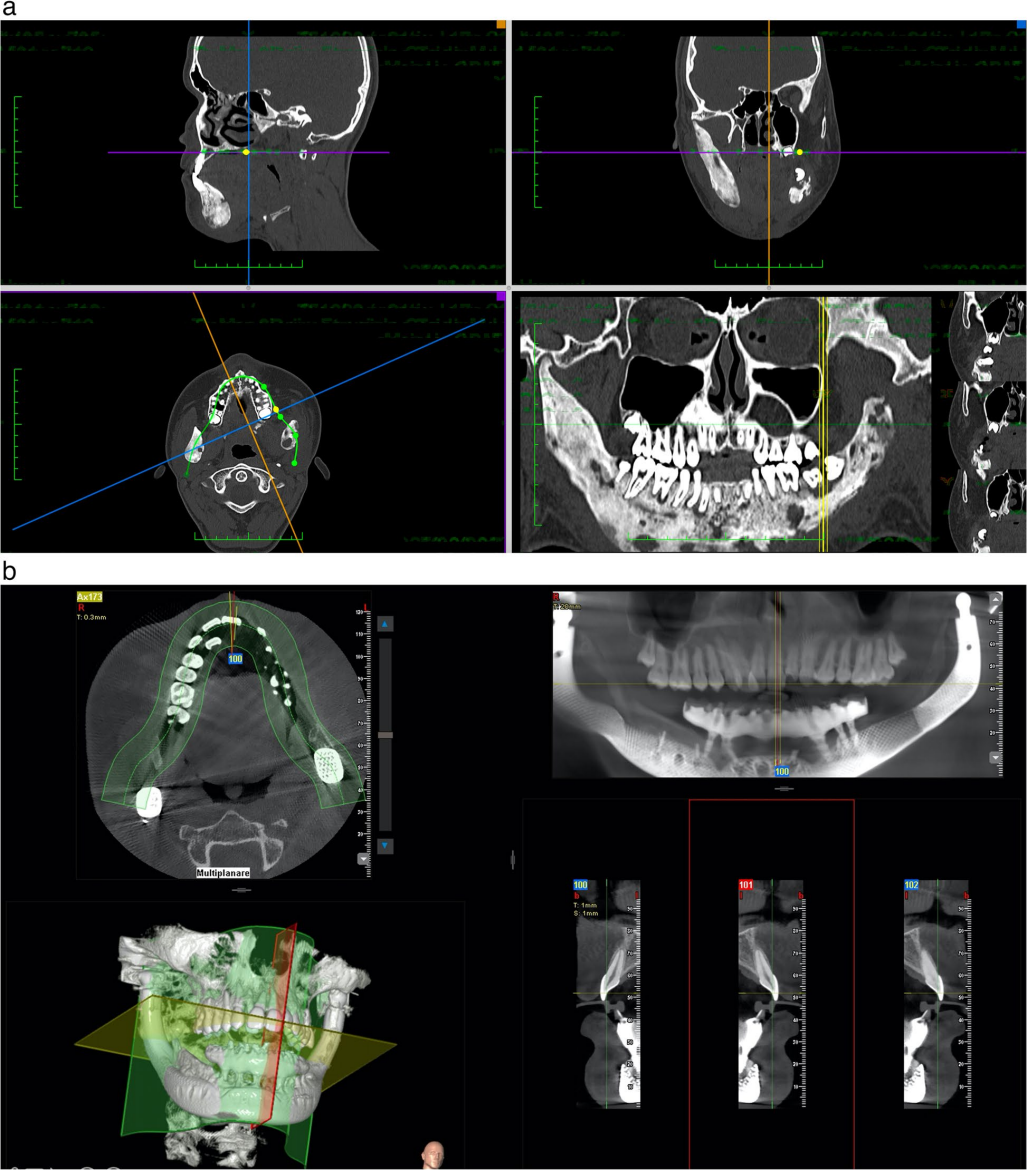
a Pre-operative CT scans. b Post-operative CT scans at the 18^th^-month follow-up after dental implant rehabilitation
